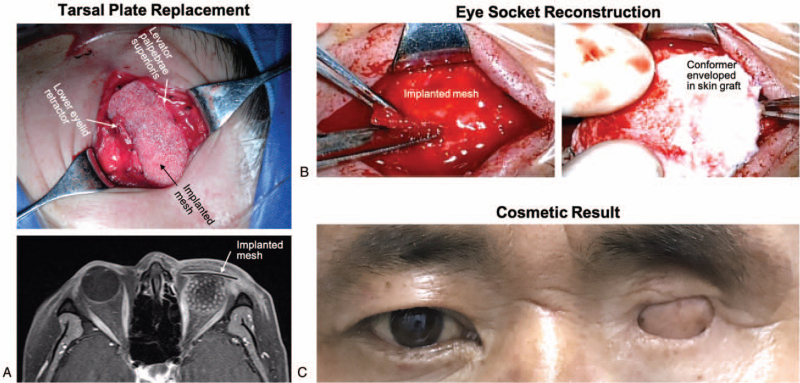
Surgical techniques for eye socket reconstruction. (A) The levator palpebrae superioris and lower eyelid retractor were sutured to a biocompatible mesh which replaced the tarsal plates. The mesh was clearly viewed on enhanced T1-weighted MRI scan. (B) The implanted mesh was cut into 2 pieces, and a conformer-enveloped dermal graft was sutured underneath to reconstruct the eye socket. (C) The final cosmetic result of eye socket reconstruction is presented.
